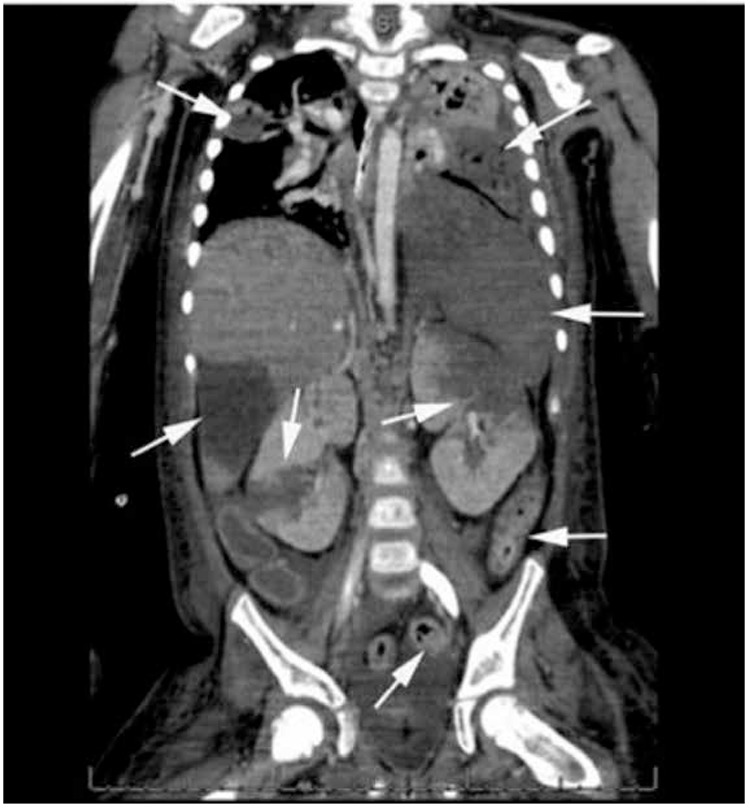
Thorax and abdominal computed tomography of the patient demonstrating bilateral areas of consolidation in the lung parenchyma and multiple infarcts in the left lower lobe of the lungs, in the liver, in the spleen, in the left upper lobe of the left and middle zone of the right kidney, and in some parts of the intestines (arrows).
